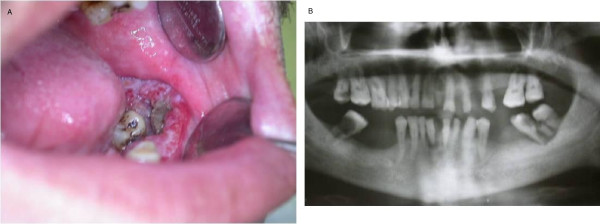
(a) Intra-oral view showing the clinical aspect of case 2. (b) Panoramic radiograph showing bone loss in the left mandibular molar region of case 2.
